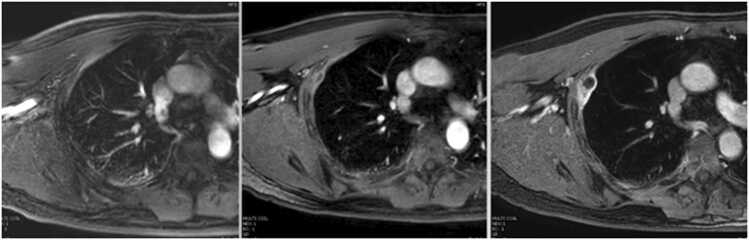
Last MRI confirmed complete resolution of the pleural hydatid cyst.
